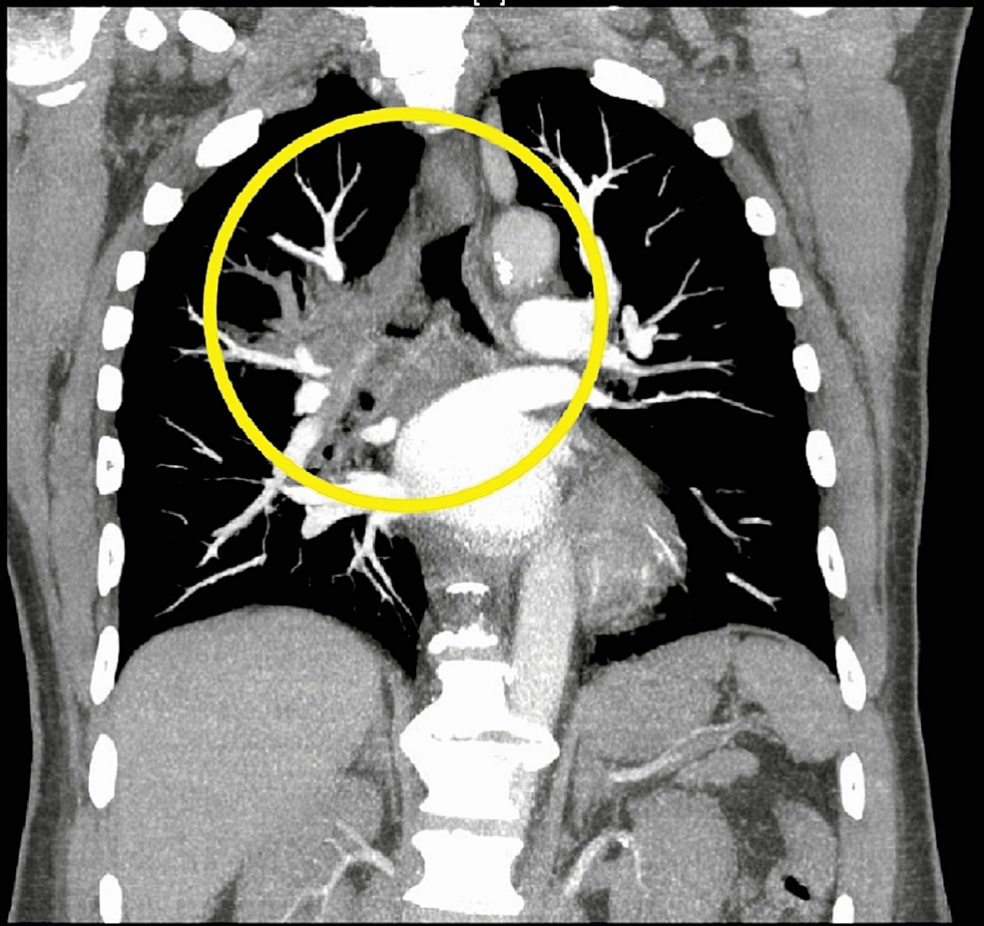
Computed Tomography Angiography of the Chest Encircled in yellow is a right hilar mass infiltrating the mediastinum measuring approximately 4.8 cm anteroposterior by 7.3 cm transverse dimensions, causing narrowing of the right bronchus and right pulmonary vasculature
